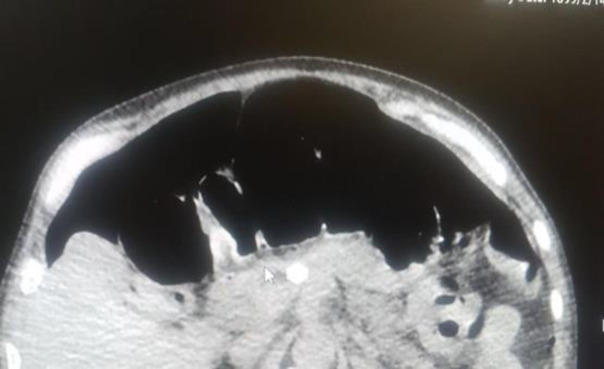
Pneumoperitoneum in CT scan
